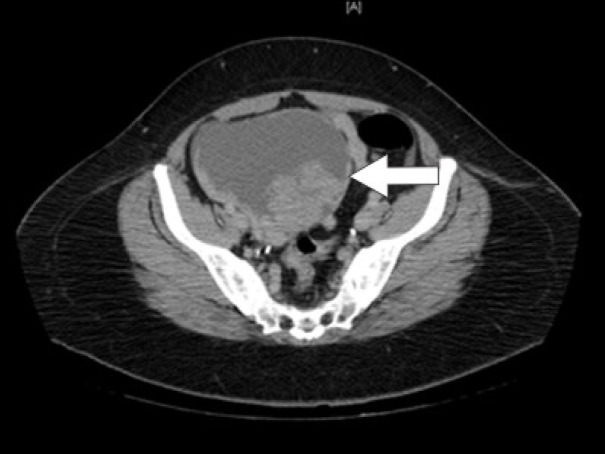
CT scan of the pelvis showing a 12.4 x 8 x 11 cm right pelvic, complex, cystic and solid appearing mass.
